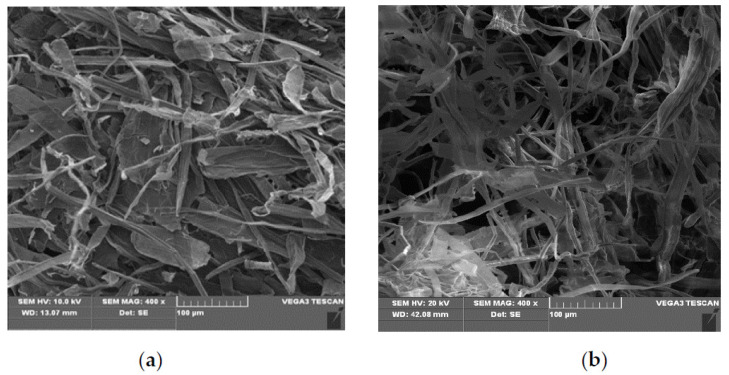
SEM images of cellulose fibers before (a) and after (b) ultrasonic irradiation.
